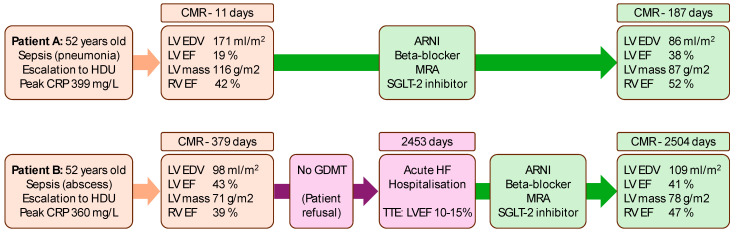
Serial cardiovascular magnetic resonance (CMR) assessments in two post-sepsis patients in relation to medical therapy. Patient A had early CMR assessment post-sepsis, which showed severely increased left ventricular (LV) end-diastolic volumes (EDV) with severely reduced LV ejection fraction (EF). Several months after guideline-directed medical therapy (GDMT) for heart failure, LV EDV was reduced, and LVEF improved. Patient B had deferred CMR assessment post-sepsis, which uncovered non-dilated LV with moderately reduced LVEF. GDMT was not commenced owing to patient refusal to take medications. The patient was hospitalised a few years later with acute heart failure decompensation with transthoracic echocardiogram (TTE) demonstrating severe LV systolic dysfunction. GDMT was commenced, and the LV function stabilised on repeat CMR within 2 months. ARNI: angiotensin receptor-neprilysin inhibitor; CRP: C-reactive protein; HDU: high dependency unit; MRA: mineralocorticoid receptor antagonist; RV: right ventricular.
